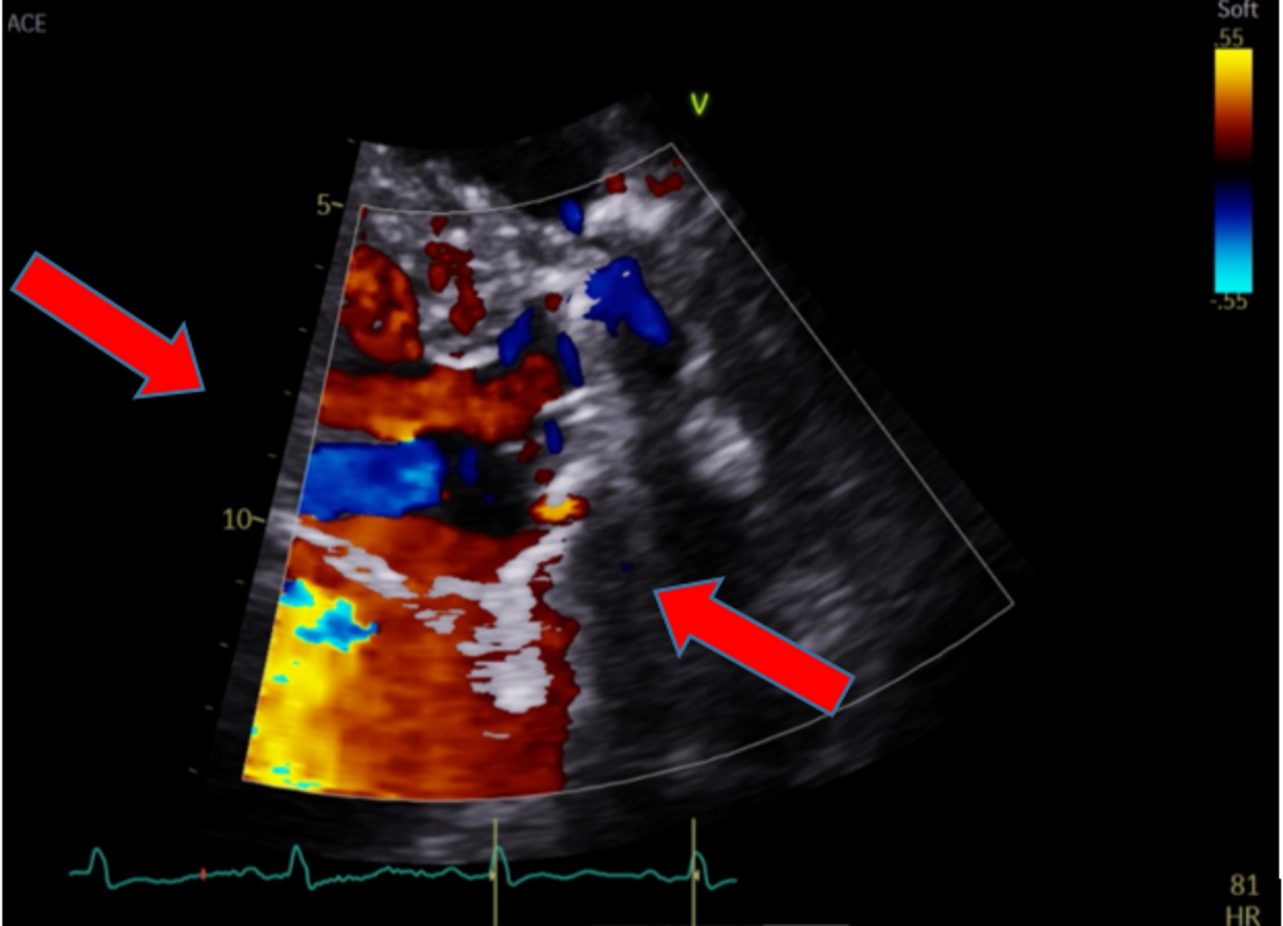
Transthoracic echocardiogram with suboptimal image quality and mild mitral regurgitation by color Doppler
